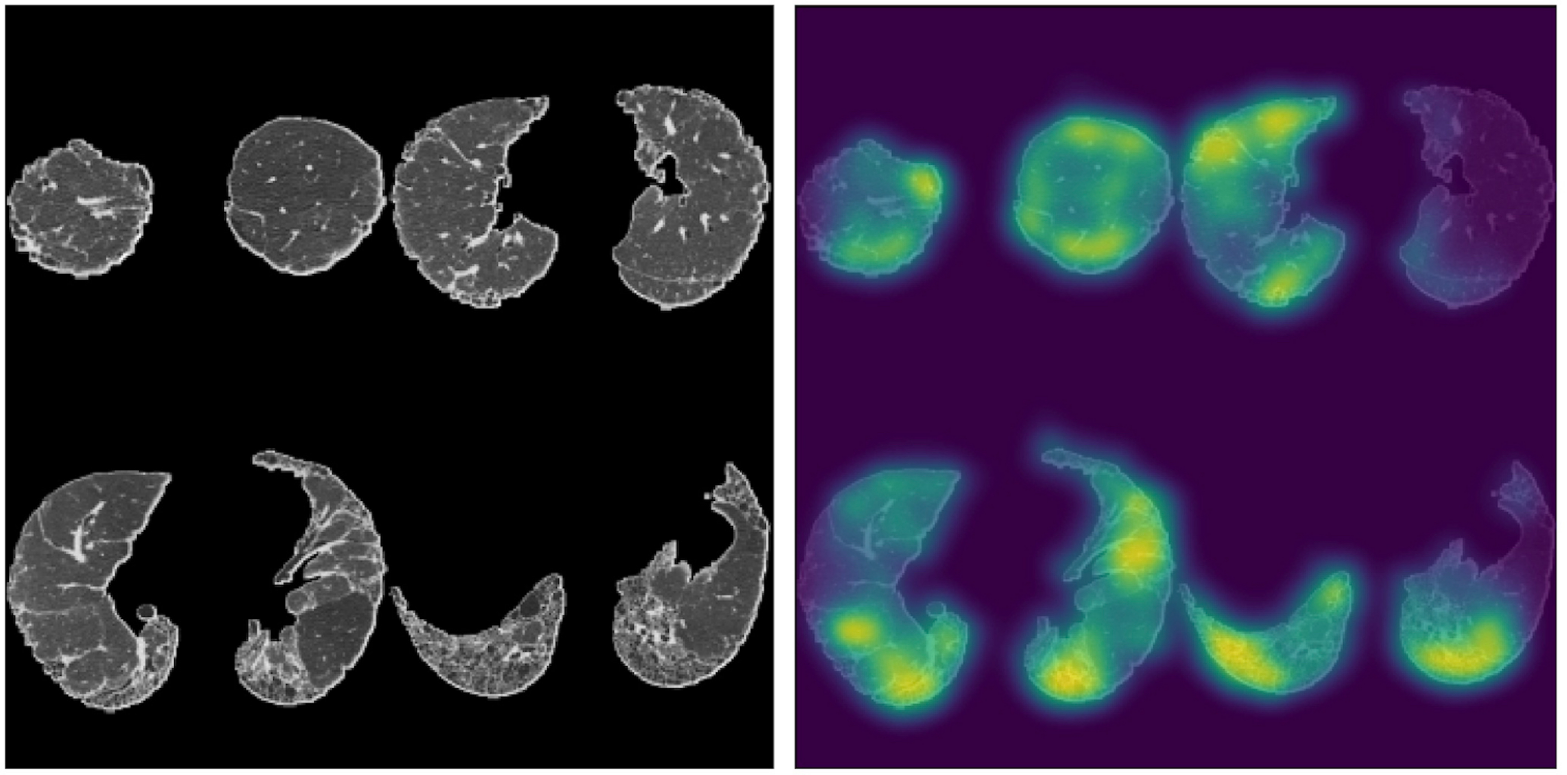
Four slice montage generated from a patient with an assigned HRCT diagnosis of probable UIP based on two expert thoracic radiologists, accompanied by a saliency map depicting parts of the lungs most influential in SOFIA-based decision-making. SOFIA probabilities for this montage were UIP: 0.224, probable UIP: 0.764, indeterminate for UIP 0.012, alternative diagnosis: 0.000. The SOFIA deep-learning algorithm generates up to 500 four axial slice montages from an HRCT scan by segmenting the lungs into quarters (excluding the apical 10%) and then randomly selecting slices from each quarter. The algorithm generates a set of numbers from 0 to 1, totalling 1.0, representing the probability of each of the UIP diagnosis categories (definite UIP, probable UIP, indeterminate, alternative diagnosis); which is the average probability for each diagnostic category across all the montages generated for each HRCT (85). Sourced and reproduced with permission from Simon L. F. Walsh, M.D., F.F.R.R.C.S.I., National Heart and Lung Institute, Imperical College London, London, United Kingdom.
